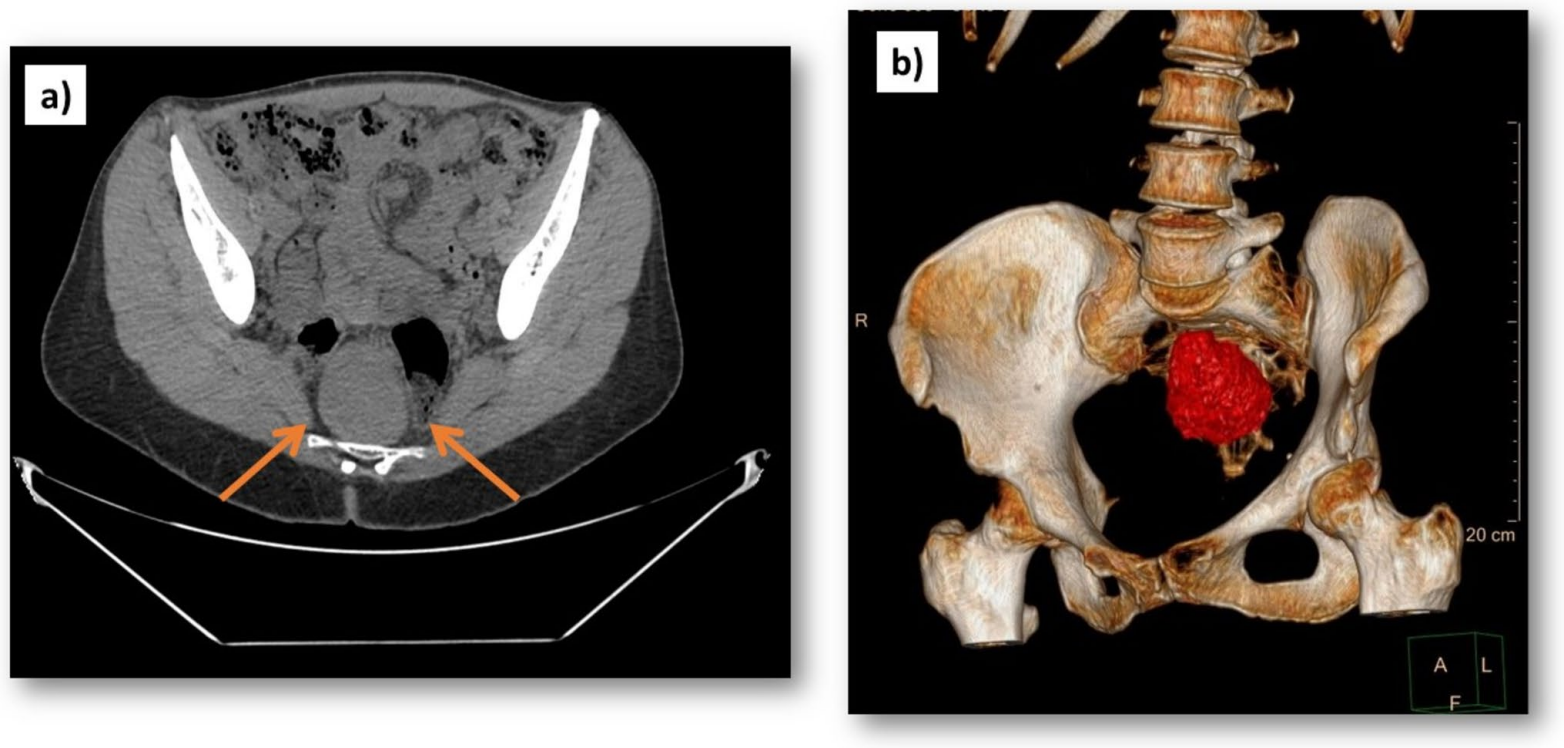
Preoperative abdomino-pelvic imaging test of a RRT: a cross sectional CT-scan; b 3D reconstruction of CT-scan
